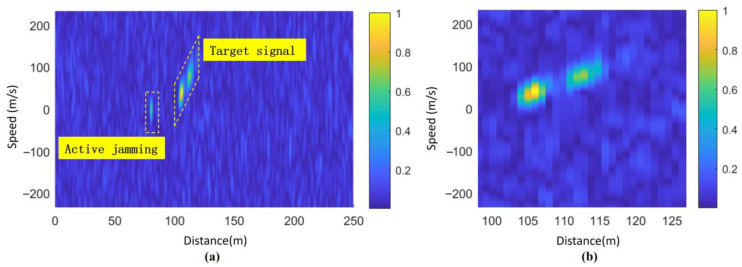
Beating signal range Doppler spectrum image: (a) full-range RD spectrum image and (b) estimated range RD spectrum image.
